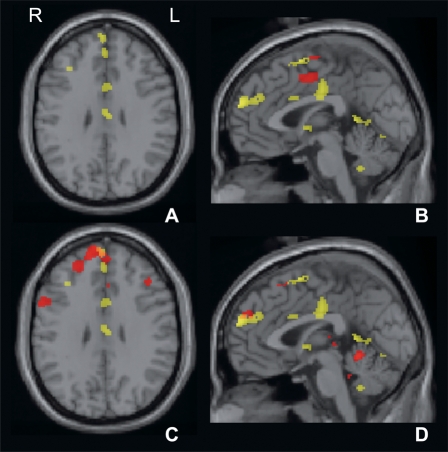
Axial (A, C) and medial sagittal slices (B, D) of the T1-weighted MNI brain with superimposed positively-loaded regions of PC 1 (red on A, B), PC 26 (red on C, D) and PC 32 (yellow on all slices). Note: (1) in the image of PC 1 the SMA and a superior dorsomedial area close to the cingulate cortex are involved exclusively; (2) in the image of PC 26 a small part of the pre-SMA and the medial-frontal cortex are implicated; and (3) in PC 32 the medial structures, i.e. the pre-SMA, the medial-frontal, the intermediate and posterior cingulate cortex, are most extensively incorporated into the network. Axial slices are at Talairach z-axis 31 mm, sagittal slices at Talairach x-axis 0.4 mm.
